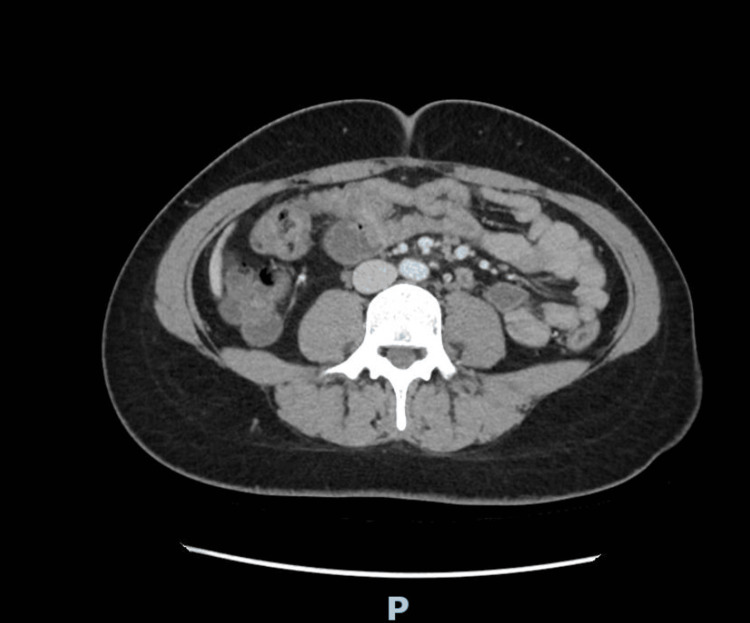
Axial contrast-enhanced CT image of the mid-abdomen. No bowel obstruction, inflammatory process, or intra-abdominal collection is seen.
